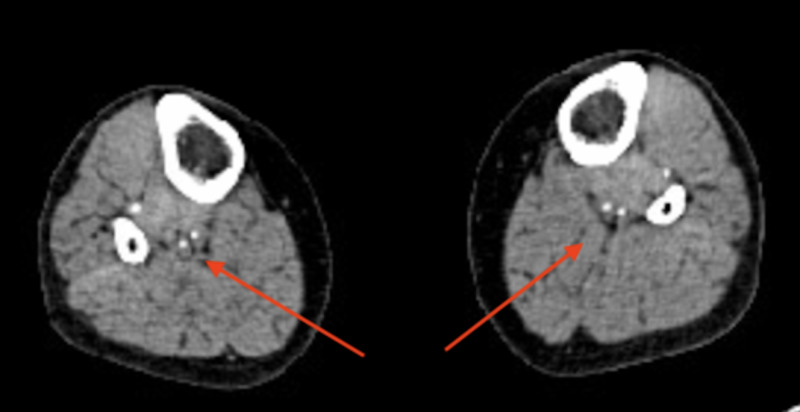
Computed tomography angiogram showing a reconstitution of flow in right anterior and posterior tibial arteries (arrows)
